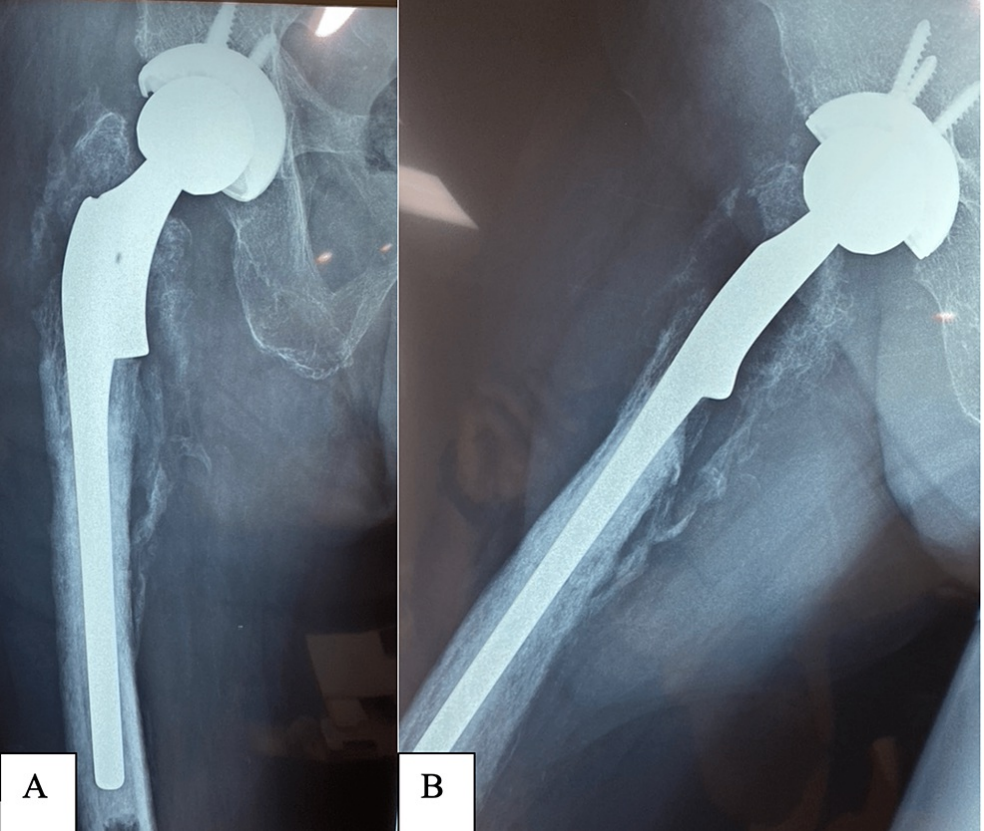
Anteroposterior (A) and lateral radiographs (B) of the total hip arthroplasty showing outline ossification of the proximal femur with formation of a neo-cortex.
